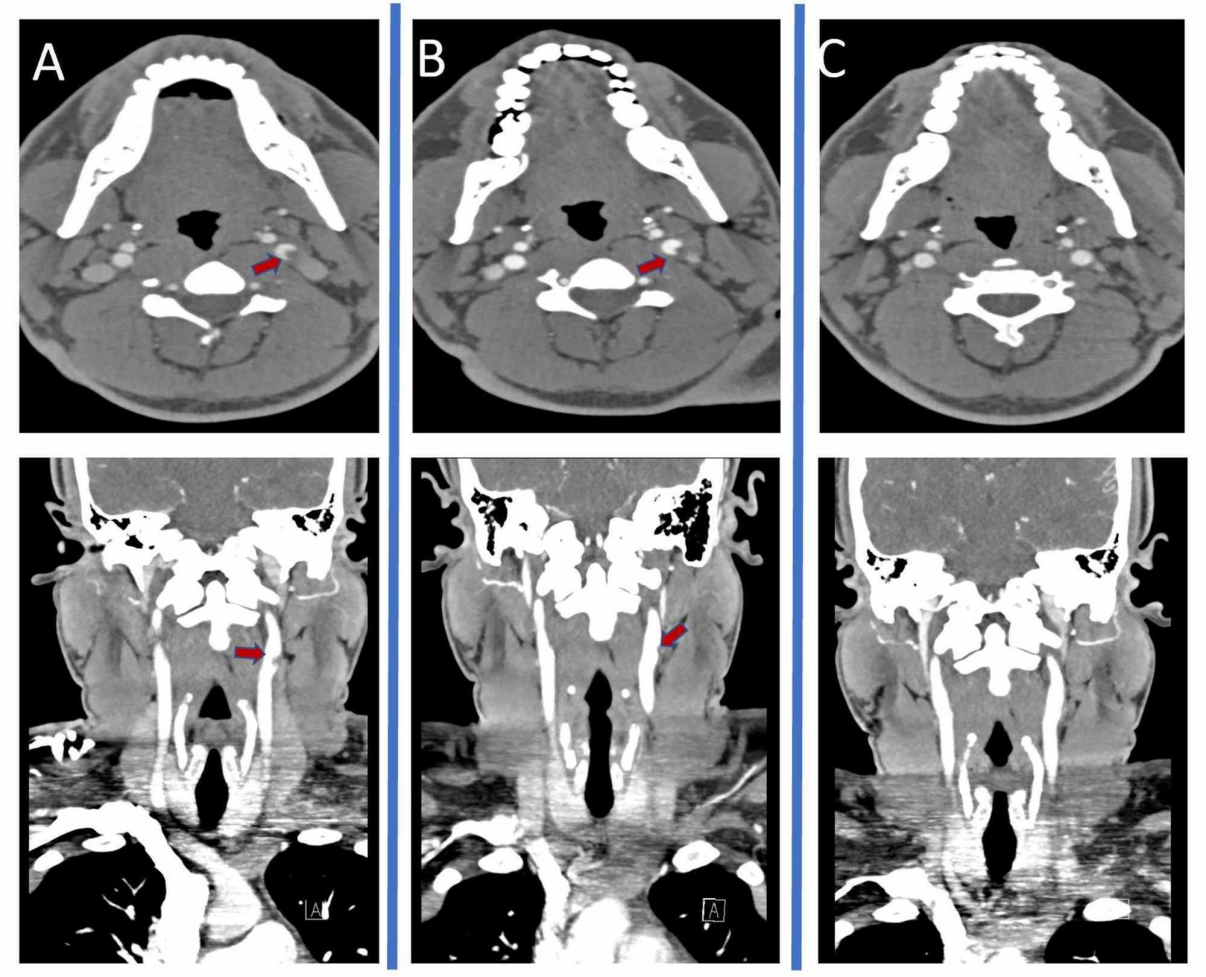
Serial CT angiography (CTA) imaging of Internal Carotid Thrombus A) Axial and coronal CTA on admission  B) Axial and coronal CTA done three days following admission  C) Axial and Coronal CTA done at discharge
